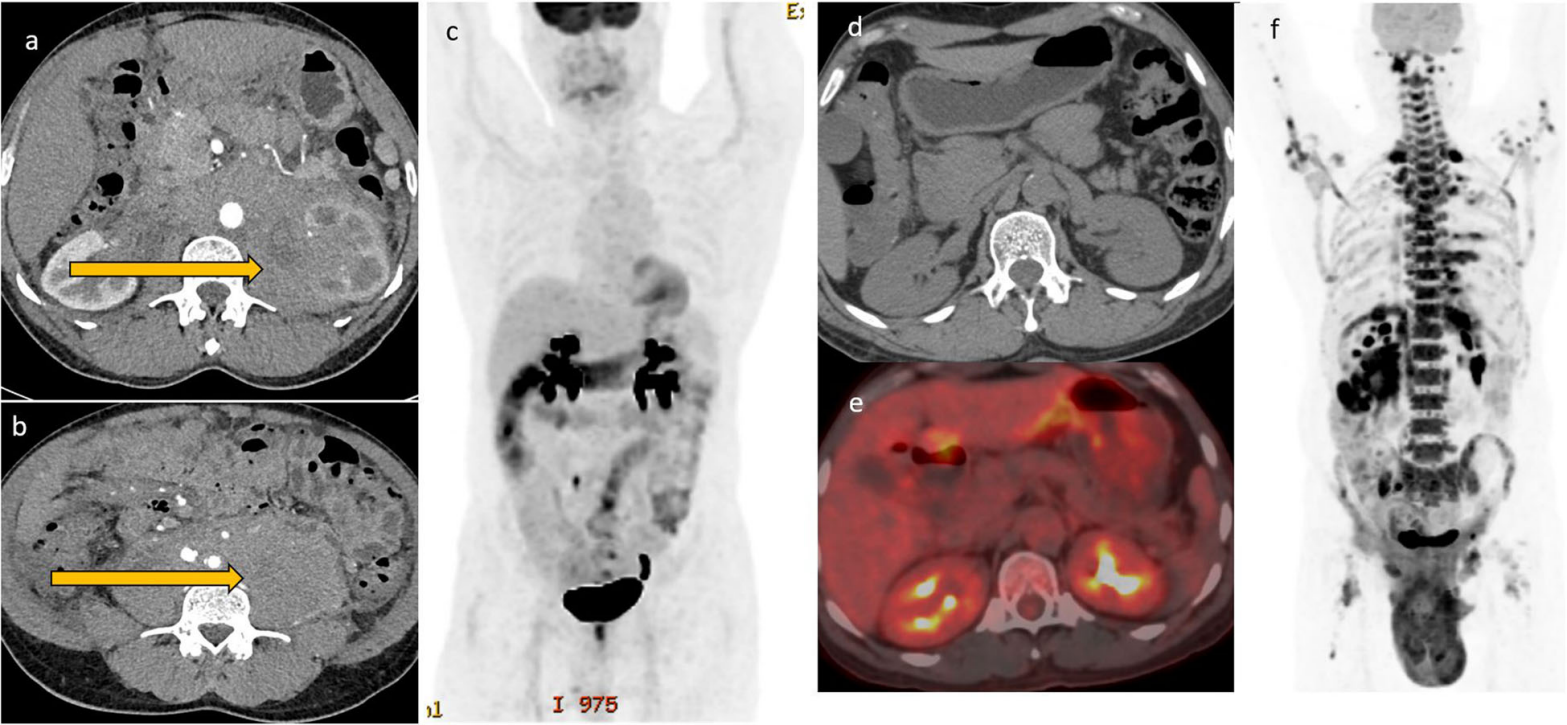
This patient with extensive retroperitoneal and renal disease (a and b) showed excellent response to treatment in the interim PET CT (c, d, and e) after two cycles of R-CHOP. There was however early relapse with bulky disease developing in the retroperitoneum and the scrotum (f)
